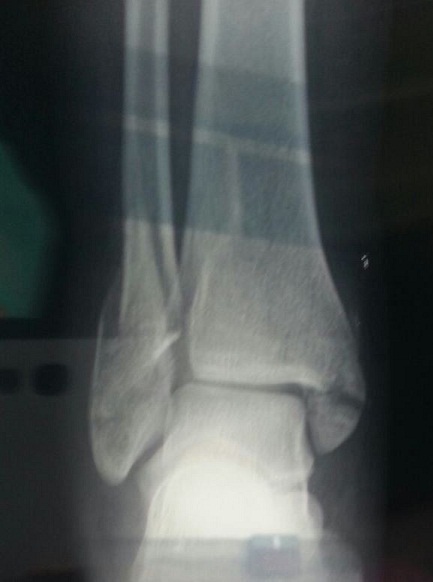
Radiographie de la cheville gauche montrant la fracture bimalléolaire
